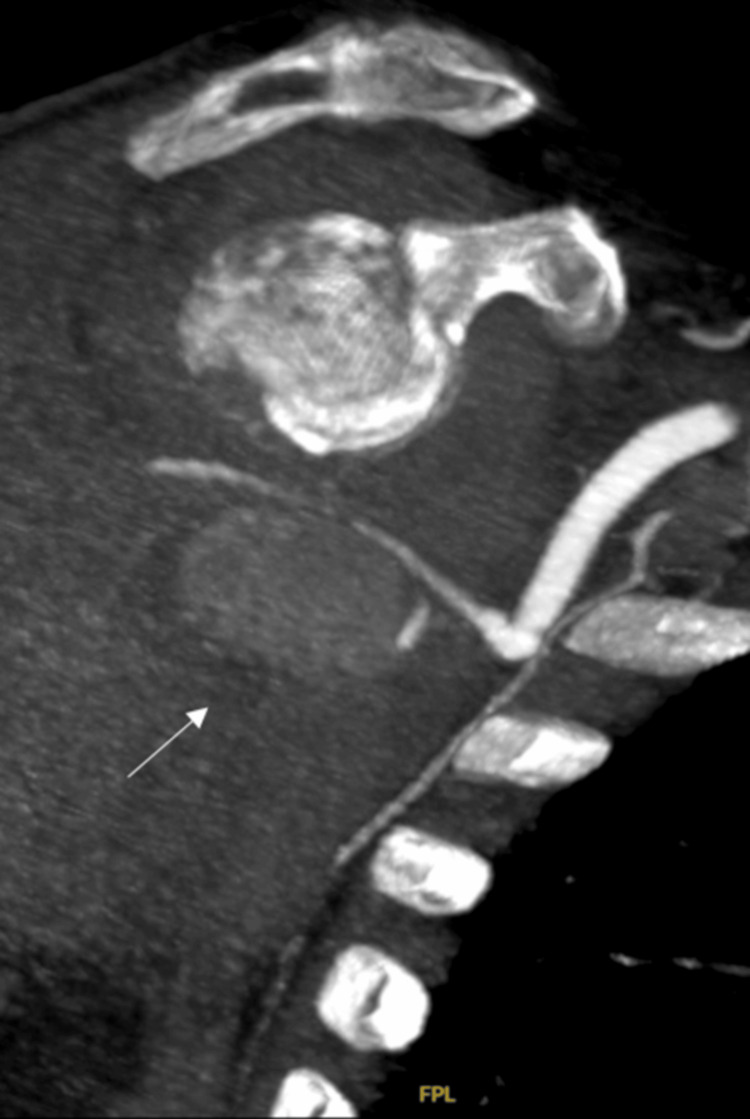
CTA showing a pseudoaneurysm of the posterior circumflex branch of the axillary artery The arrow indicates the pseudoaneurysm. CTA: computed tomography angiogram
